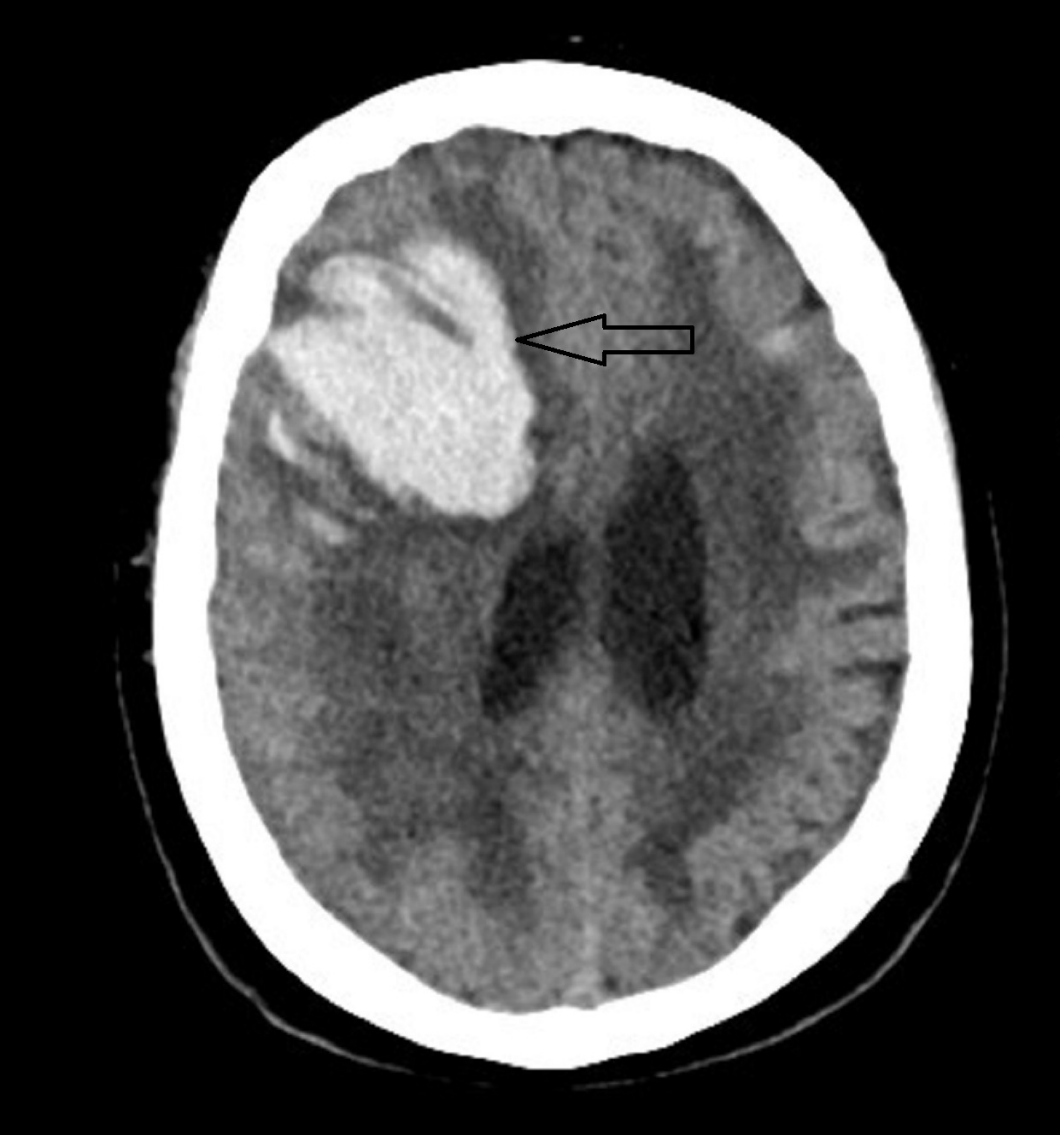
Initial Computed Tomography (CT) Head From Outside Hospital CT shows a right frontal intracerebral hemorrhage (56.5 cc) with mass effect on the right lateral ventricle.
